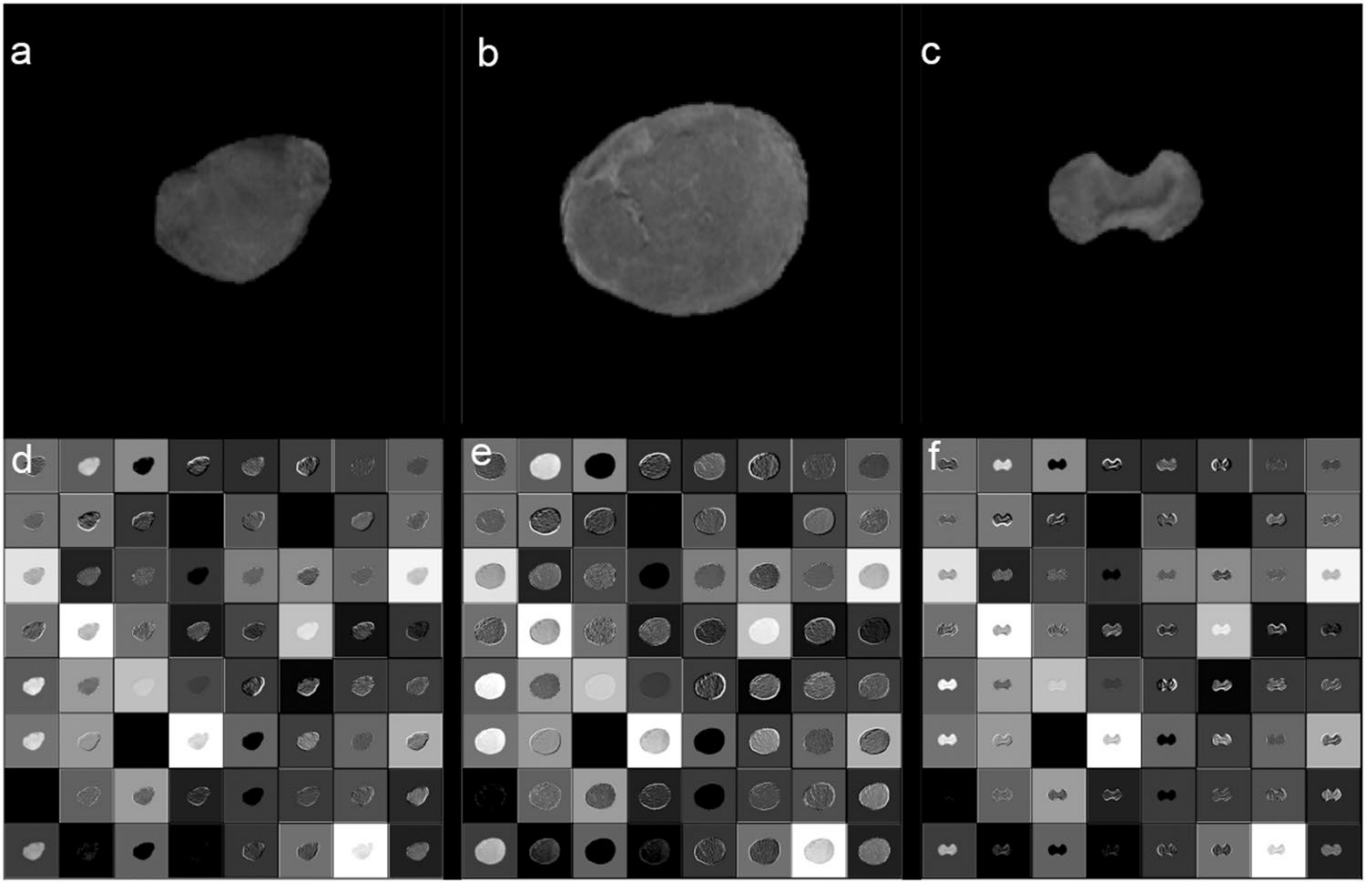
The visualization of features maps from the first convolutional layer of the cervical tumor at superior (a, d), middle (b, e), and inferior level (c, f) on T2-weighted MR images. Notice that the model exhibits responses to low-level visual elements such as edges and textures. These elements are crucial as they serve as foundational building blocks for recognizing complex patterns within images. Edges often represent boundaries between different objects or regions, potentially identifying the morphological characteristics of tumors in this case. Textures may provide information about tumor heterogeneity. By combining these basic elements, our model could capture more complex patterns associated with tumors.
